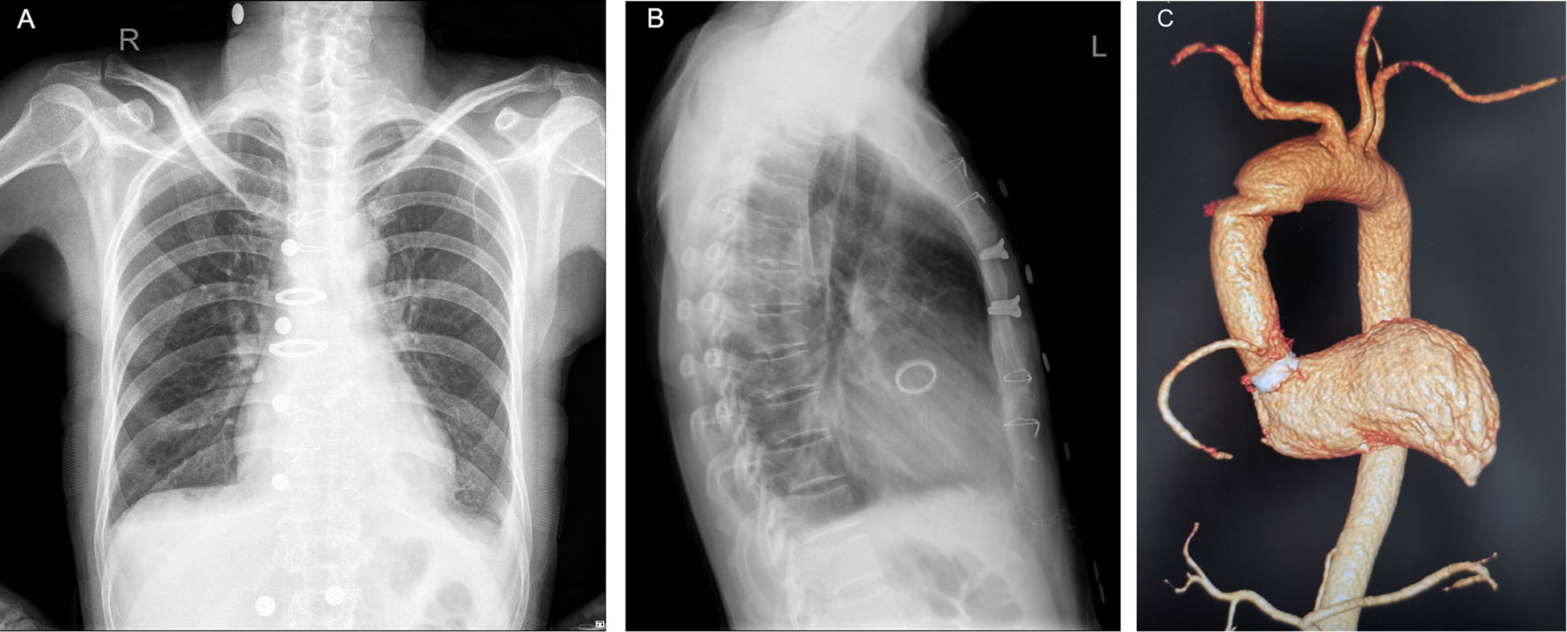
Postoperative anteroposterior (A) and lateral (B) chest X-rays indicate that the aorta is basically normal after Bentall procedure. Chest CTA (C) showed that the artificial vessel and coronary arteries were normal and unobstructed after Bentall procedure
